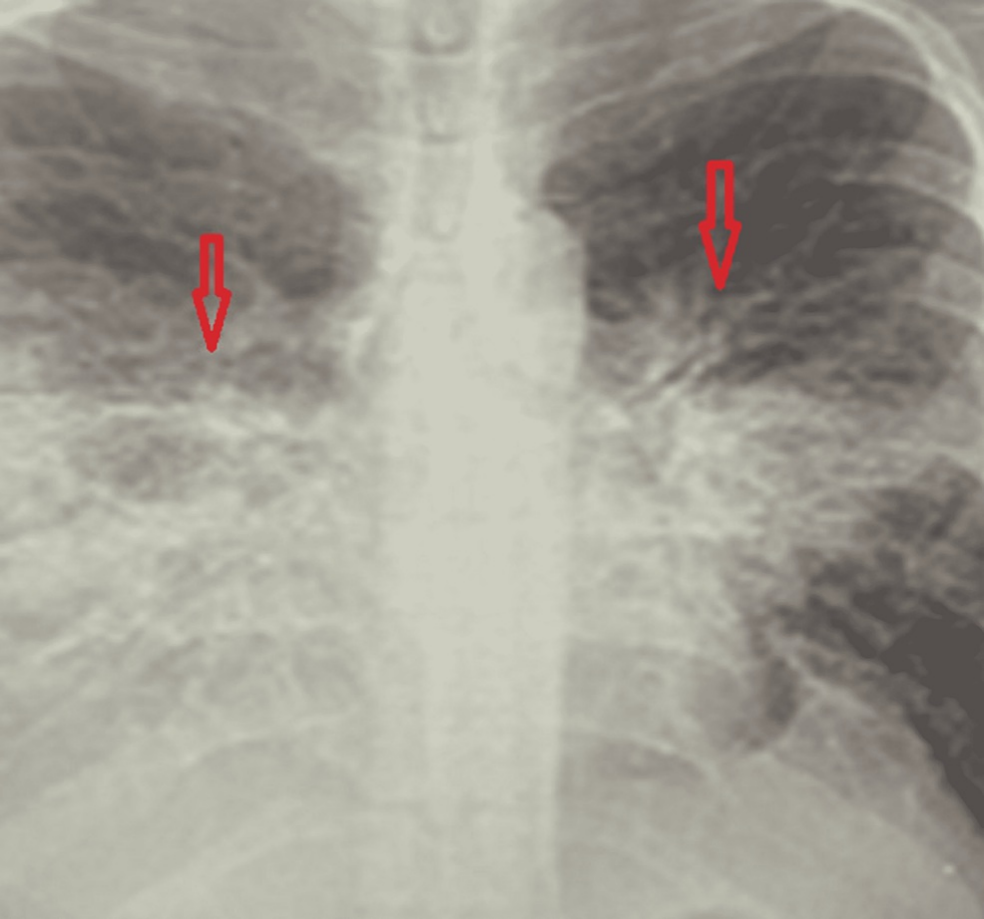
CXR revealing diffuse perihilar interstitial opacities (bilateral vertical arrows) and bilateral pleural effusion worse on the right side CXR: Chest x-ray.
